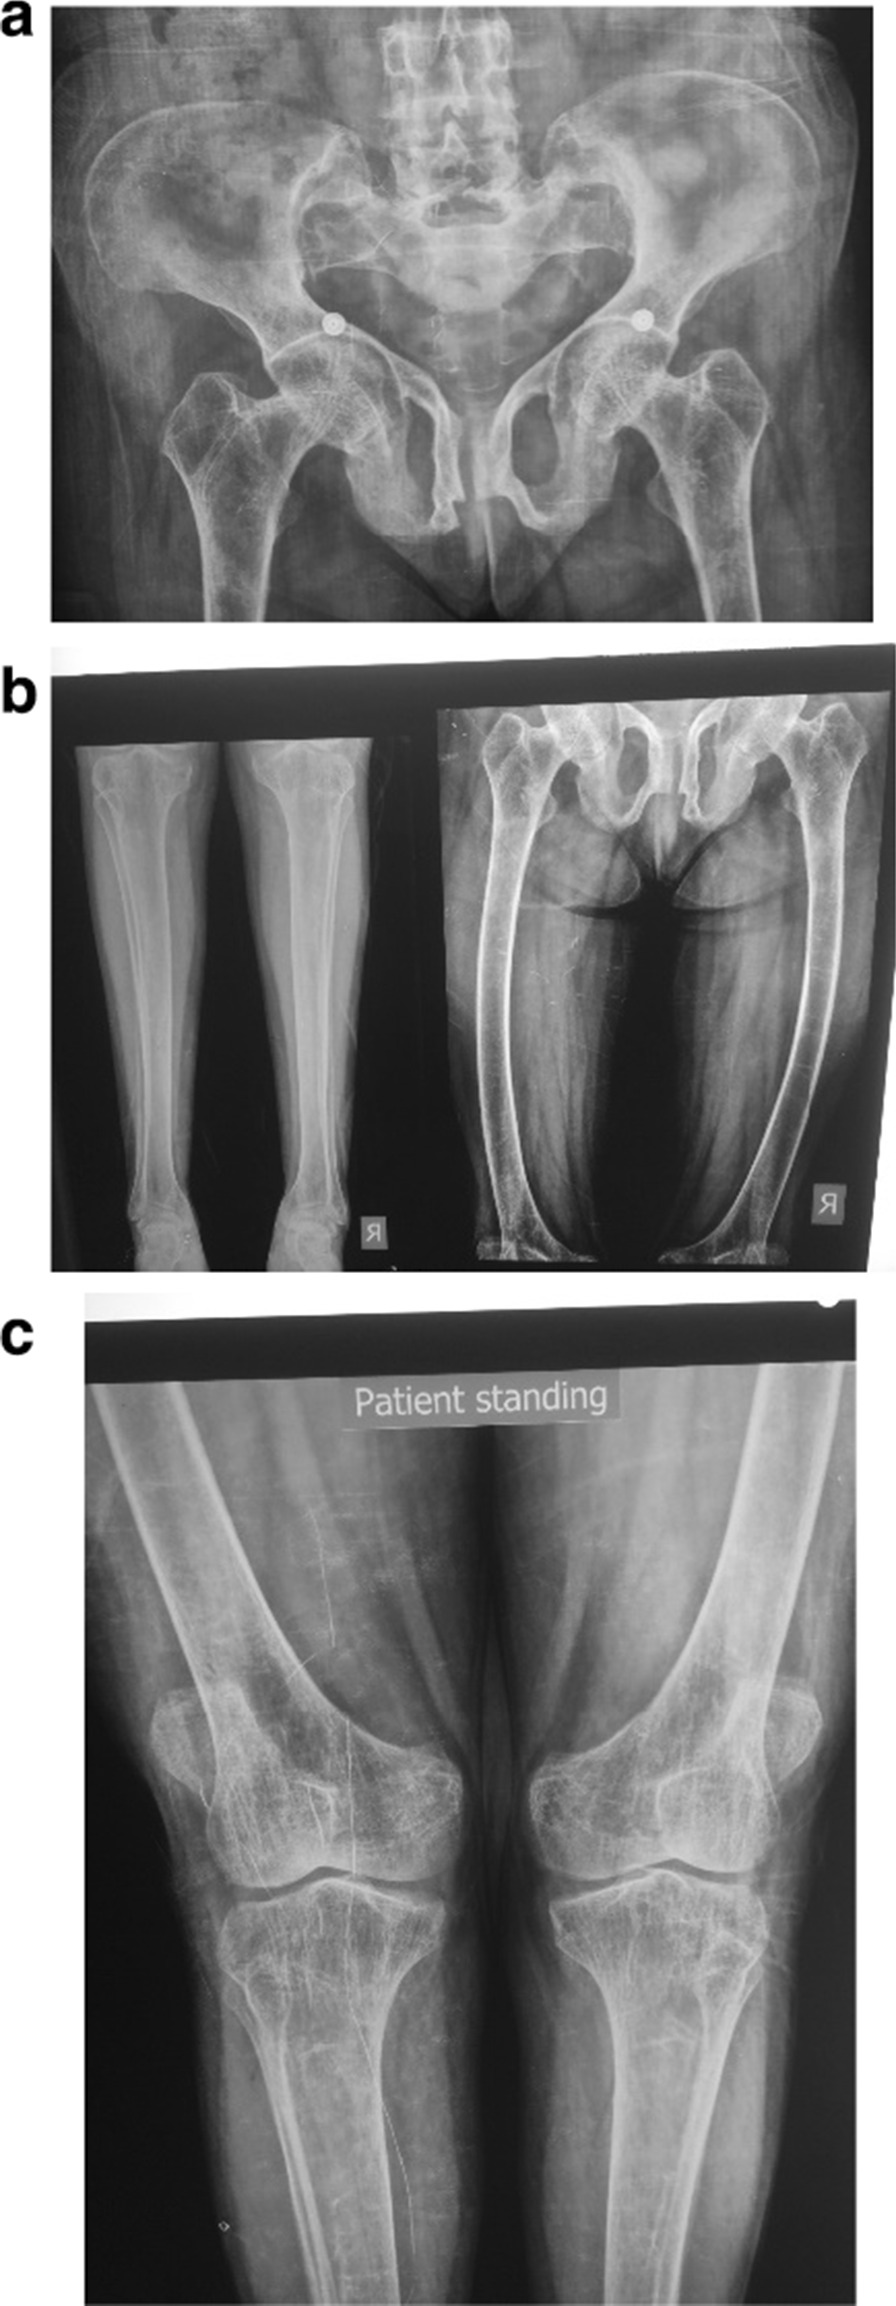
a Pelvic X-ray. Osteopenia around hip joints. Abnormal shaped pelvic brim and abnormal shaped pubic bones and acetabular fossa. Preserved joint spaces of both hips. b Leg X-rays. Bowing of both femurs, and mild osteopenia mainly around ankle joints. c Knee X-rays. Osteopenia mainly around knee joints. Bowing of both femurs and bilateral genu valgus deformity and prominent epicondyles
